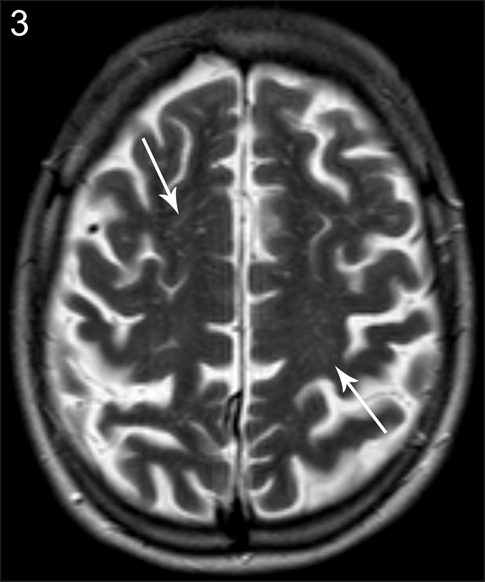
Patient A, axial T2-weighted image shows prominent Virchow-Robin spaces bilateral in the centrum semiovale.
